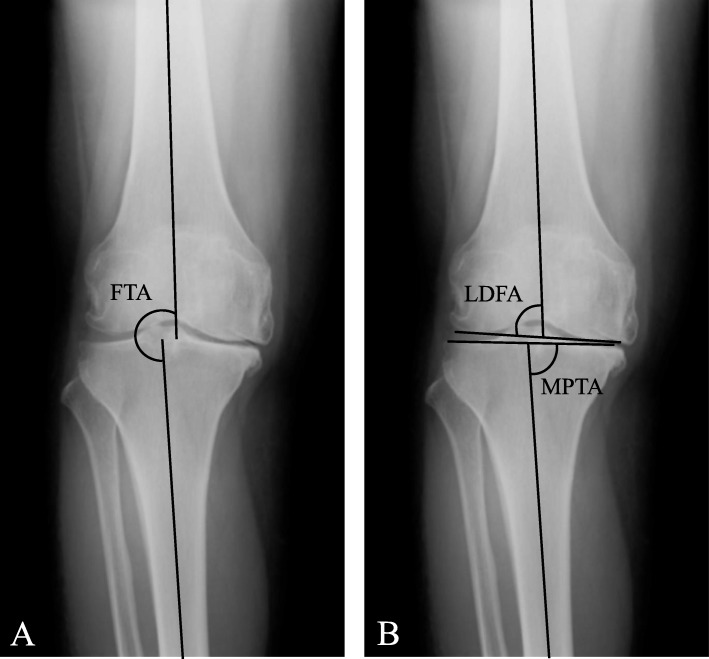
Radiographic measurement of femorotibial angle (FTA, a), medial proximal tibial angle (MPTA, b), and lateral distal femoral angle (LDFA, b) on an anteroposterior x-ray view of the knee
